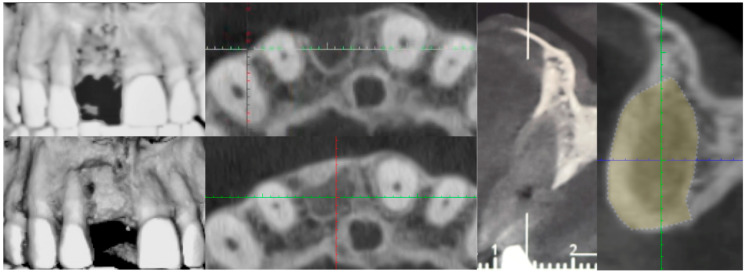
CBCT Comparison before and after augmentation. In yellow the new volume.
